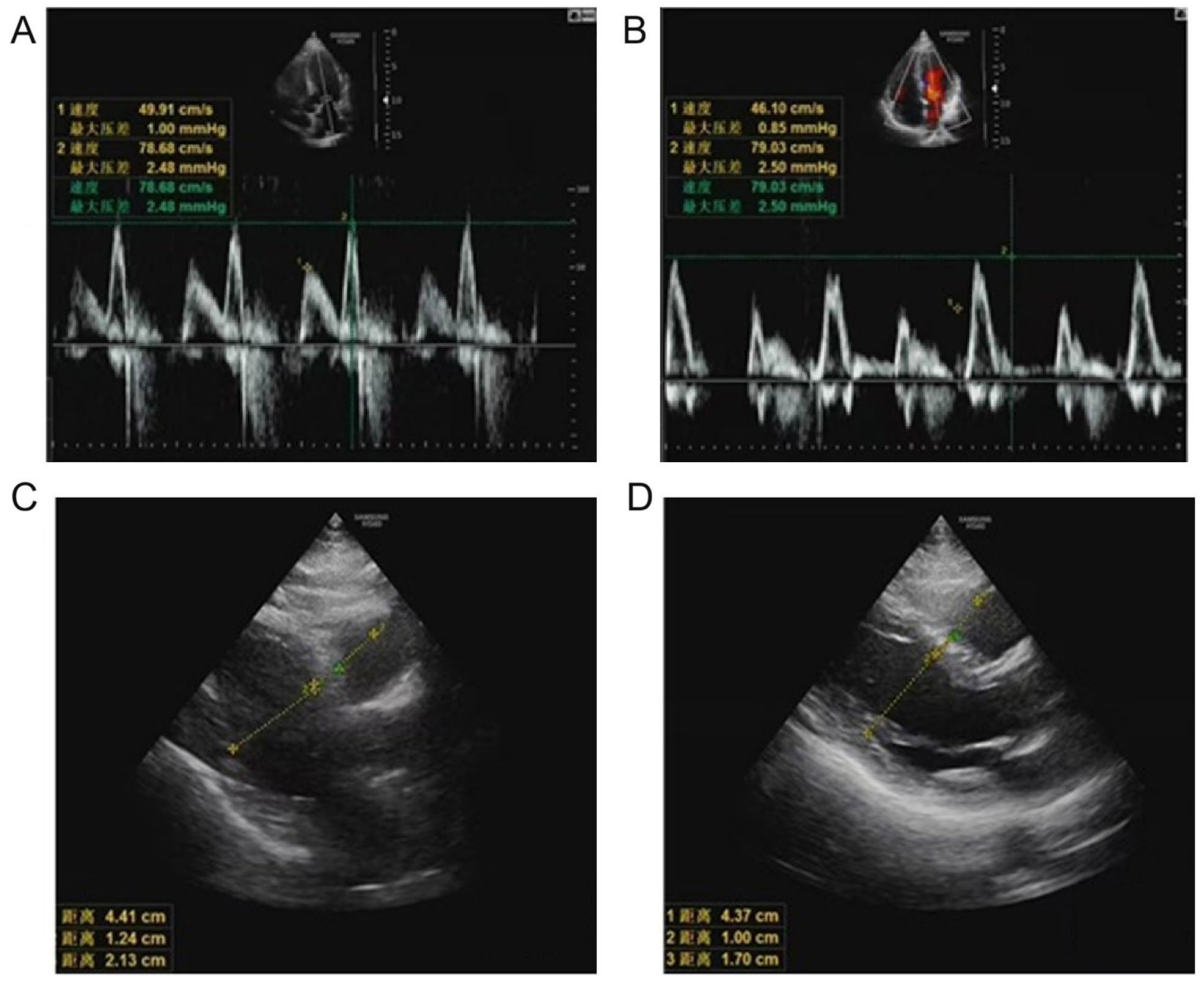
Doppler echocardiography images in women with pregnancy-induced hypertension syndrome (PIHS). (A) Mitral inflow velocity spectrum in a PIHS patient with an adverse pregnancy outcome. (B) Mitral inflow velocity spectrum in a PIHS patient with a favorable pregnancy outcome. (C) Left ventricular and left atrial diameters and wall thickness in a PIHS patient with an adverse outcome. (D) Left ventricular and left atrial diameters and wall thickness in a PIHS patient with a favorable outcome.
